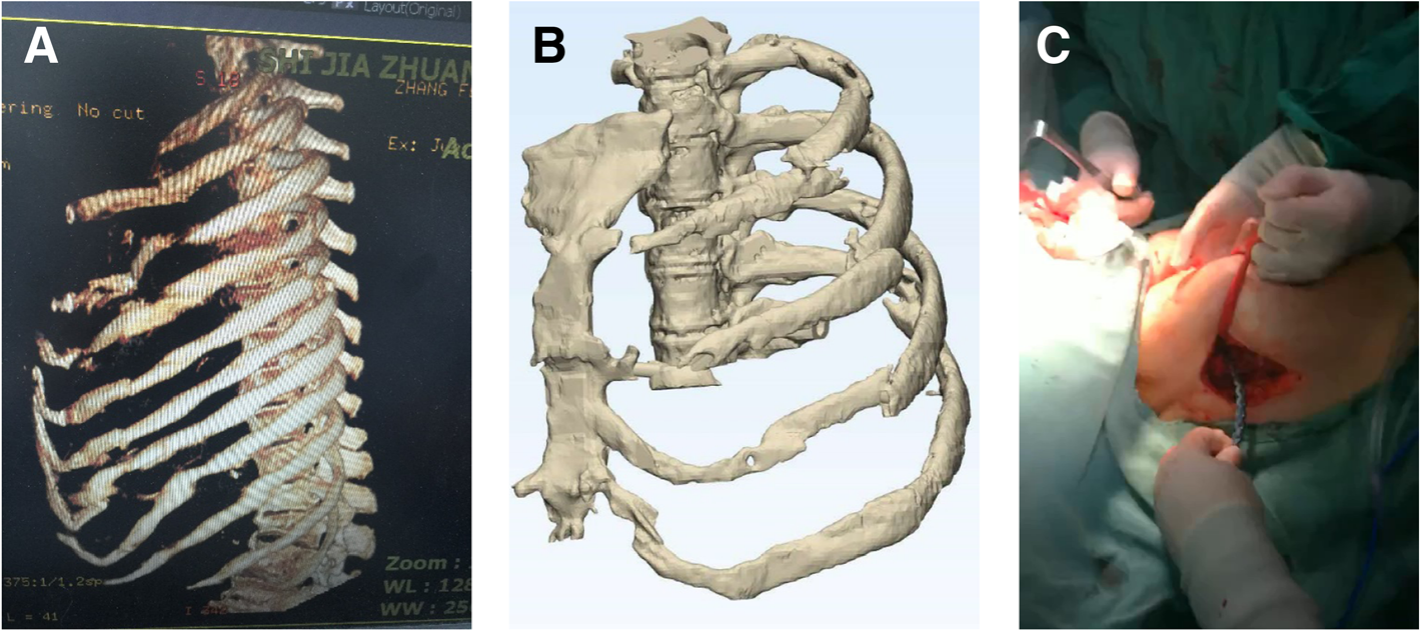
No.3 patient (a) Preoperative scanning revealed 2–11 left rib fractures where 2–6 ribs contained the costal cartilage multiple fractures involving the costal arch. b Preoperative 3–5 rib models were prepared by 3D printing technology based on CT thin slice scanning. c During the surgery, corresponding pre-shaping locking plates were placed in each rib
